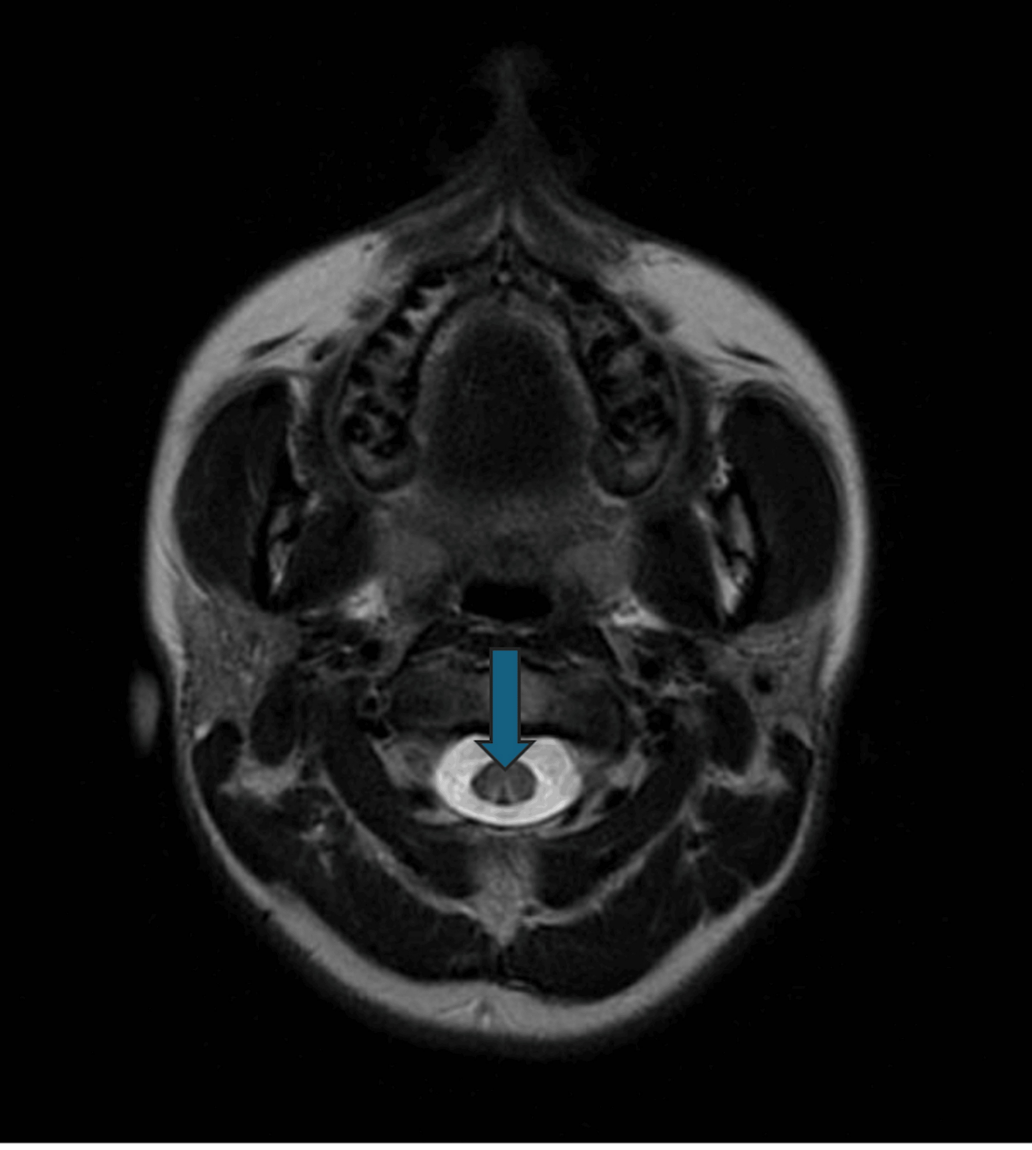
MRI of the spinal cord showing "inverted V sign." The axial T2 view of the spinal cord displays the classic "inverted V sign." This finding is characteristic of SCD, where the demyelination affects the dorsal columns symmetrically, creating this distinctive V-shaped pattern on axial imaging (blue arrow). SCD, subacute combined degeneration
